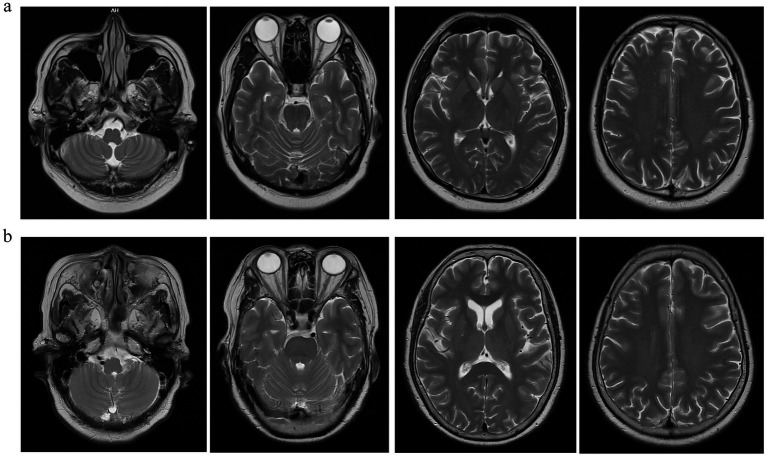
Brain MRI examination images of two cases. (a) Brain MRI images of Case 1. (b) Brain MRI images of Case 2.
